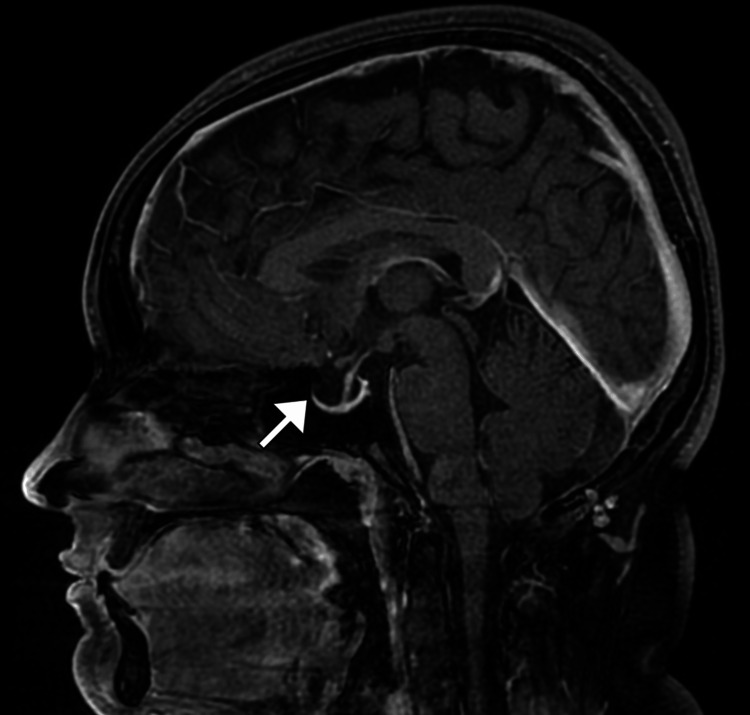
Nearly empty sella on contrast-enhanced T1-weighted MRI Sagittal contrast-enhanced T1-weighted magnetic resonance image (MRI) demonstrates an almost empty sella turcica (arrow), with flattening of the pituitary gland against the sellar floor. This appearance is a recognized radiologic sign of chronically elevated intracranial pressure and supports the diagnosis of idiopathic intracranial hypertension in the appropriate clinical context.
